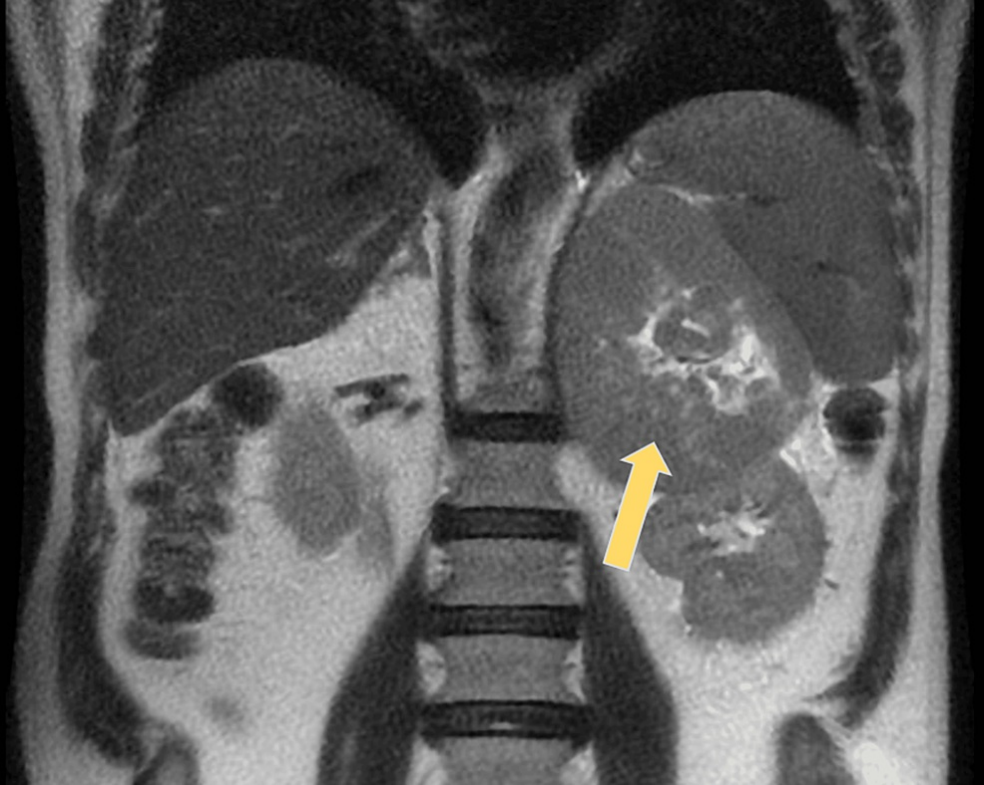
Abdominal magnetic resonance imaging The yellow arrow indicates the adrenal mass.
